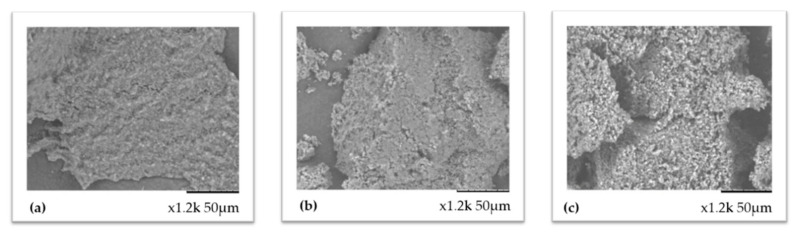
Nannochloropsis* biomass analysis using SEM: (a) Initial time; (b) ultrasound pretreatment using US bath Elmasonic S 40H at 37 kHz for 6 h at 55 °C without enzymes; (c) combined US-assisted enzymatic pretreatment using US bath Elmasonic S 40H at 37 kHz for 6 h and 55 °C in combination with Alcalase, Viscozyme, and Celluclast commercial enzymes (together).
